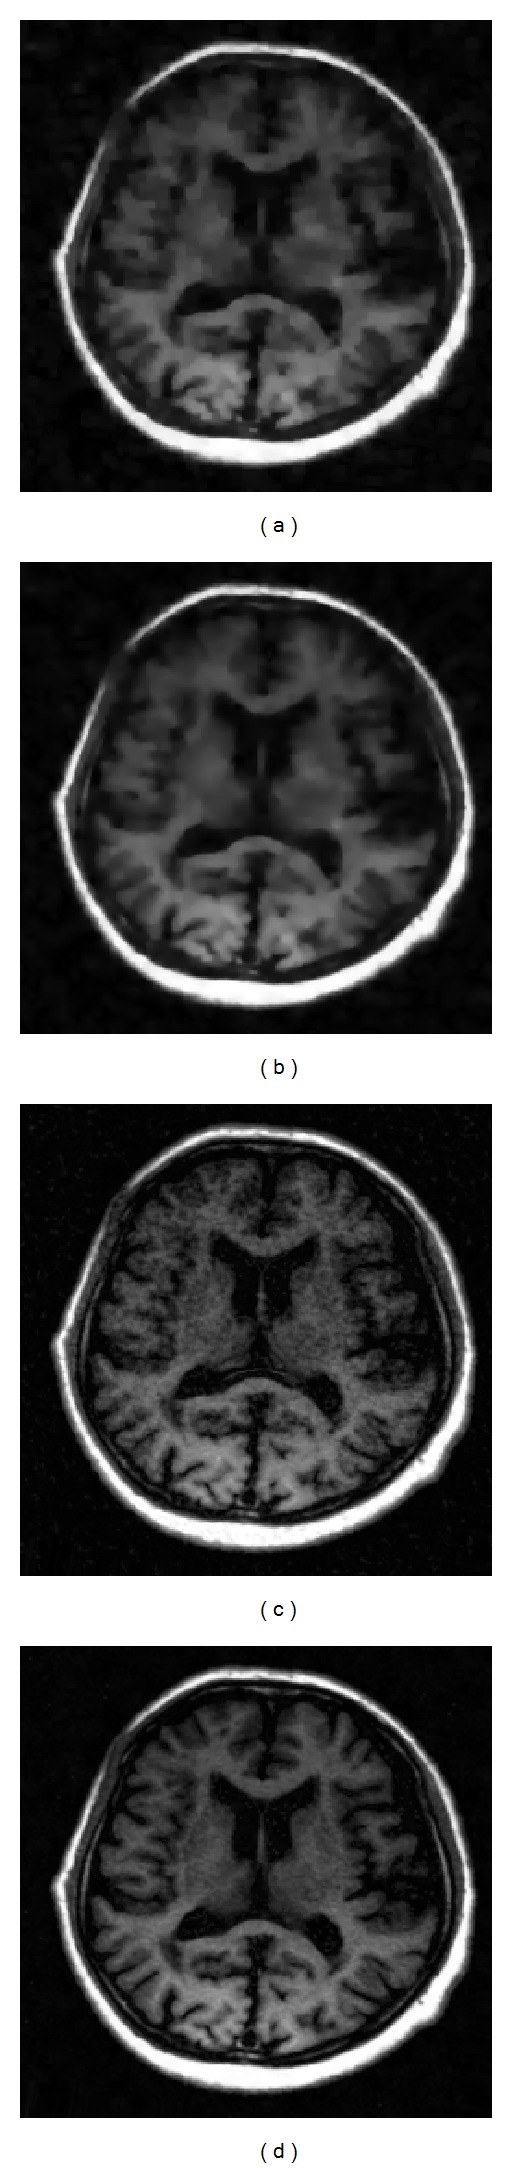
Reconstruction results with 15% of the fully sampled data by sparse-MRI (a), RL-WSTGV (b), sparse-Id (c), and the proposed method (d).
